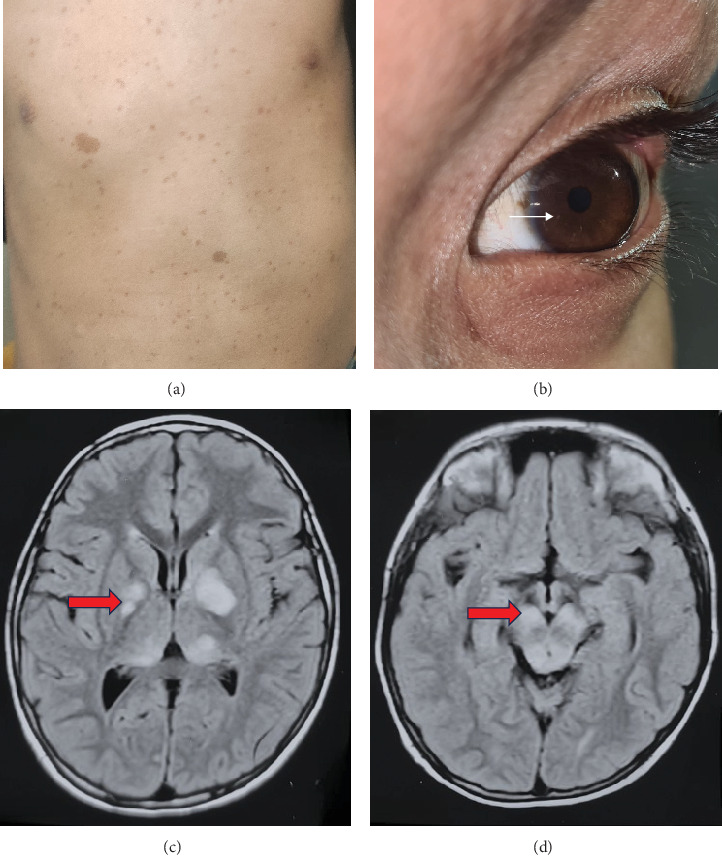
Clinicoradiological features of NF-1. Café-au-lait spots over the (a) chest and abdomen and (b) Lisch nodules seen over the iris. The MRI brain FLAIR axial section shows the focal areas of signal intensity (FASIs) indicated by red arrows in the (c) basal ganglia and thalamus and in the (d) brainstem.
